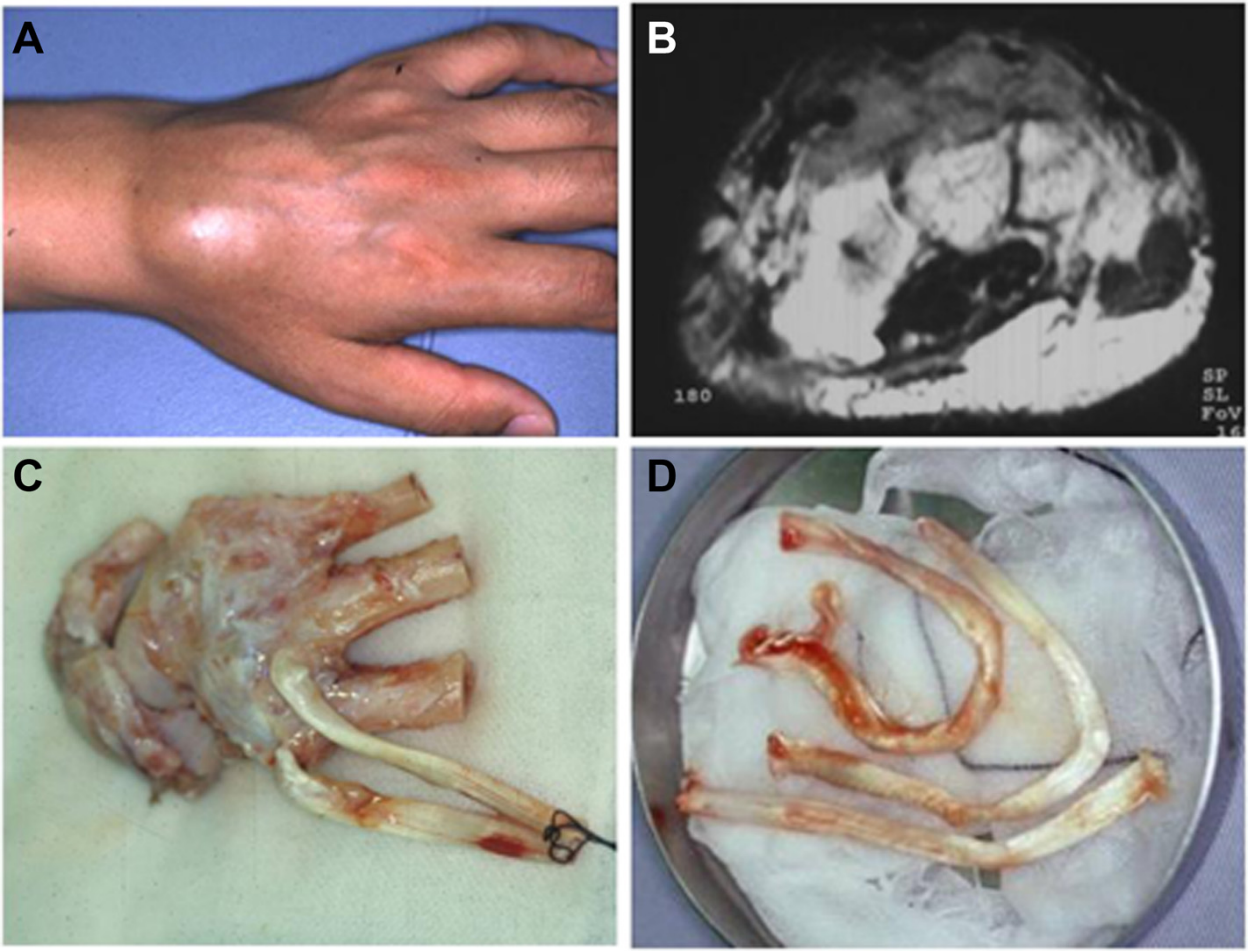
A 43-year-old male patient with clear cell sarcoma on dorsum of wrist. Clear cell sarcoma on dorsum of wrist. (A) A 43-year-old man with a 2-year history of a slow-growing mass on the dorsum of the left hand was shown. (B) MRI of the tumor was highly inhomogeneous on T2-weighted images, which was located beneath the extensor tendons and was attached to the carpal and metacarpal bones. (C, D) The tumor was resected including the carpal and metacarpal bones and extensor tendons. The resected tissues were trimmed and irradiated with 50 Gy one fraction.
